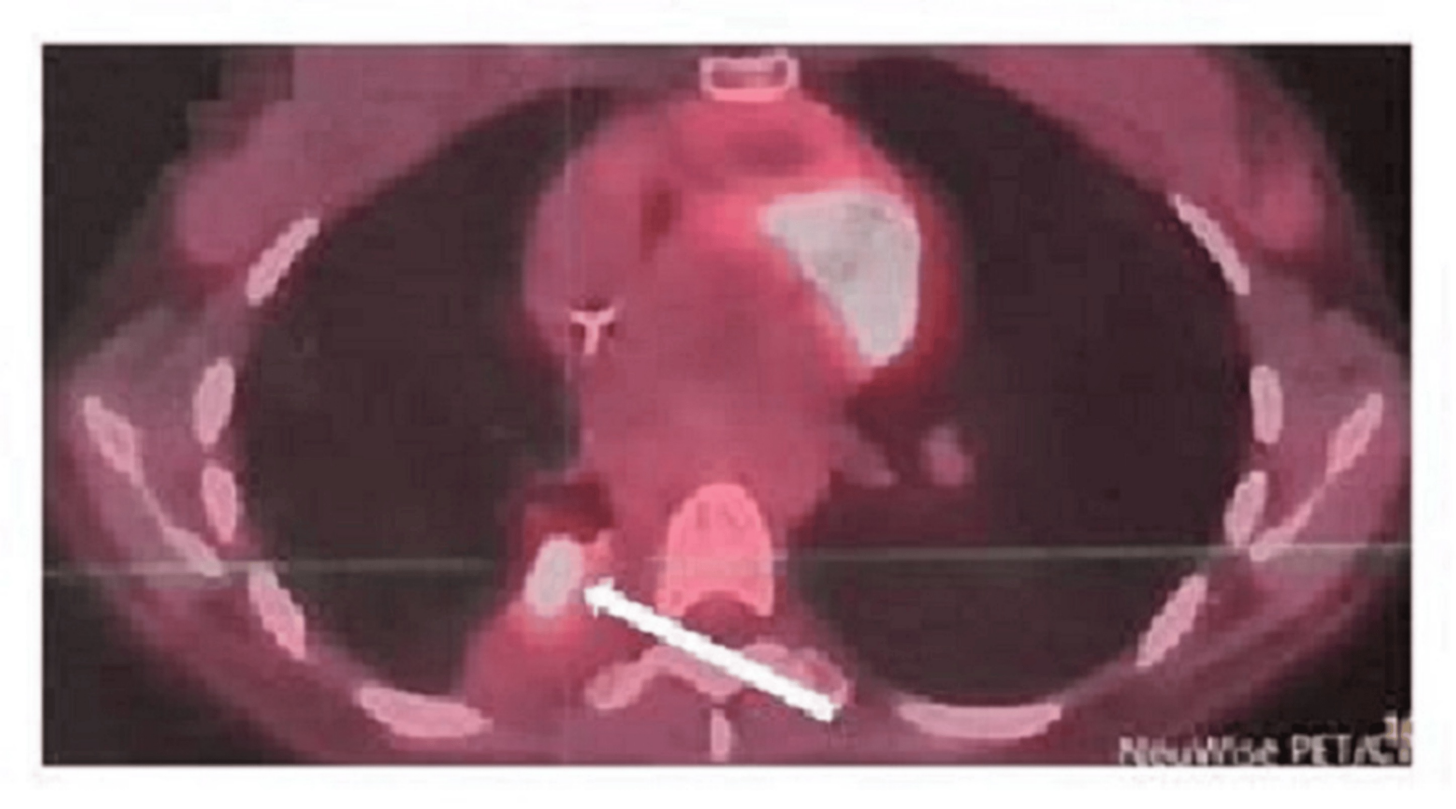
PET/CT scan before chemotherapy indicating hypermetabolic pleuropulmonary lymphomatous dissemination (white arrow).
